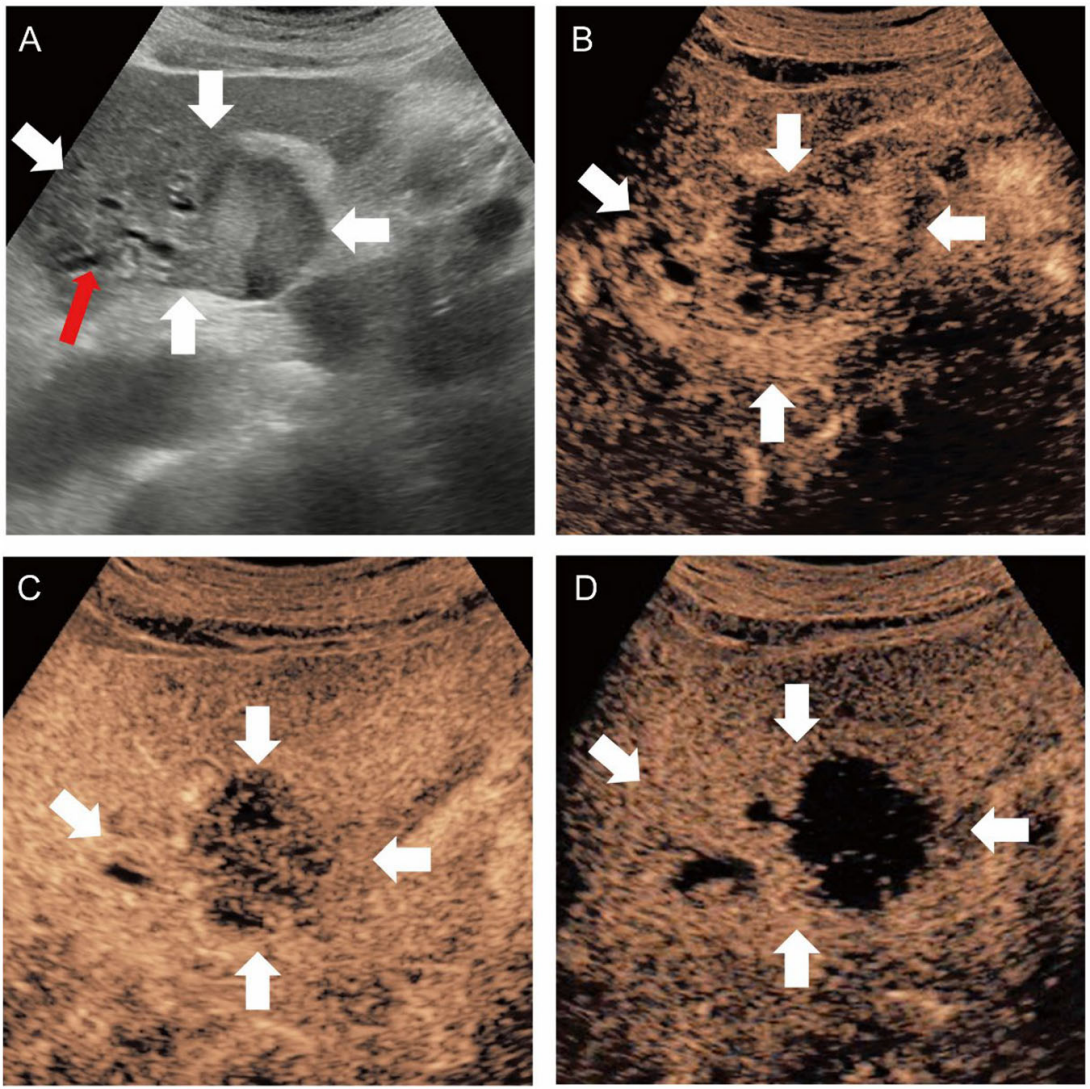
A 70-year-old male with a 32-mm biliary type MF-ICC. B-mode ultrasound showed hypo-echogenicity lesion and dilated bile duct (A). Circular enhancement was observed in the arterial phase (B). Hypo-enhancement was observed in the portal venous phase (C) and late phase (D). White arrow indicated the margin of the lesion; red arrow indicated the dilated bile duct
